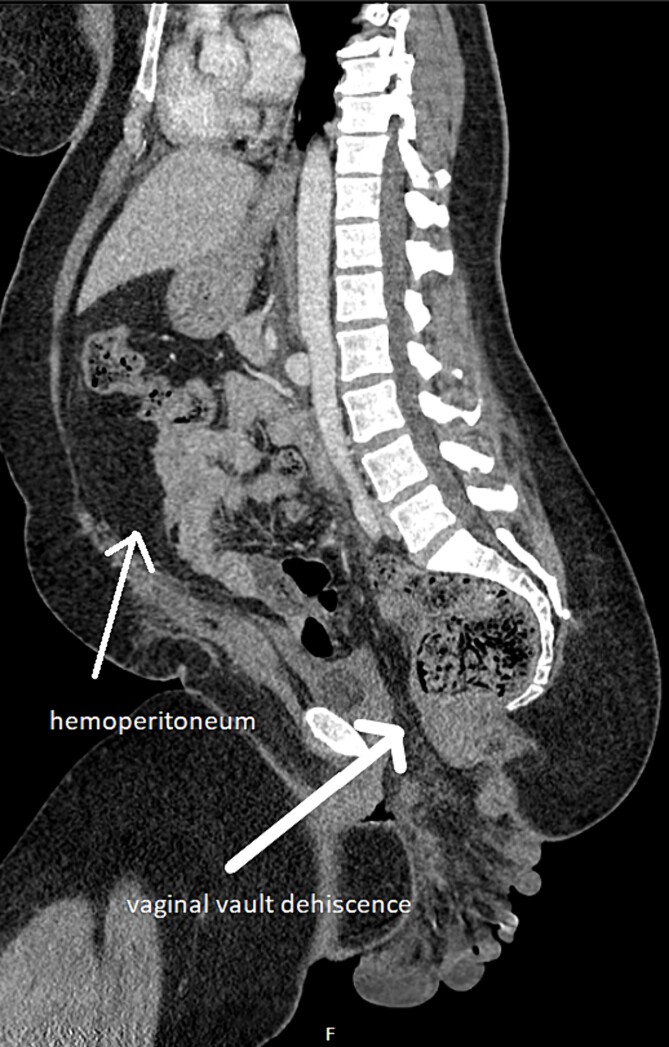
Small bowel evisceration through vaginal vault dehiscence and haemoperitoneum on CT.
